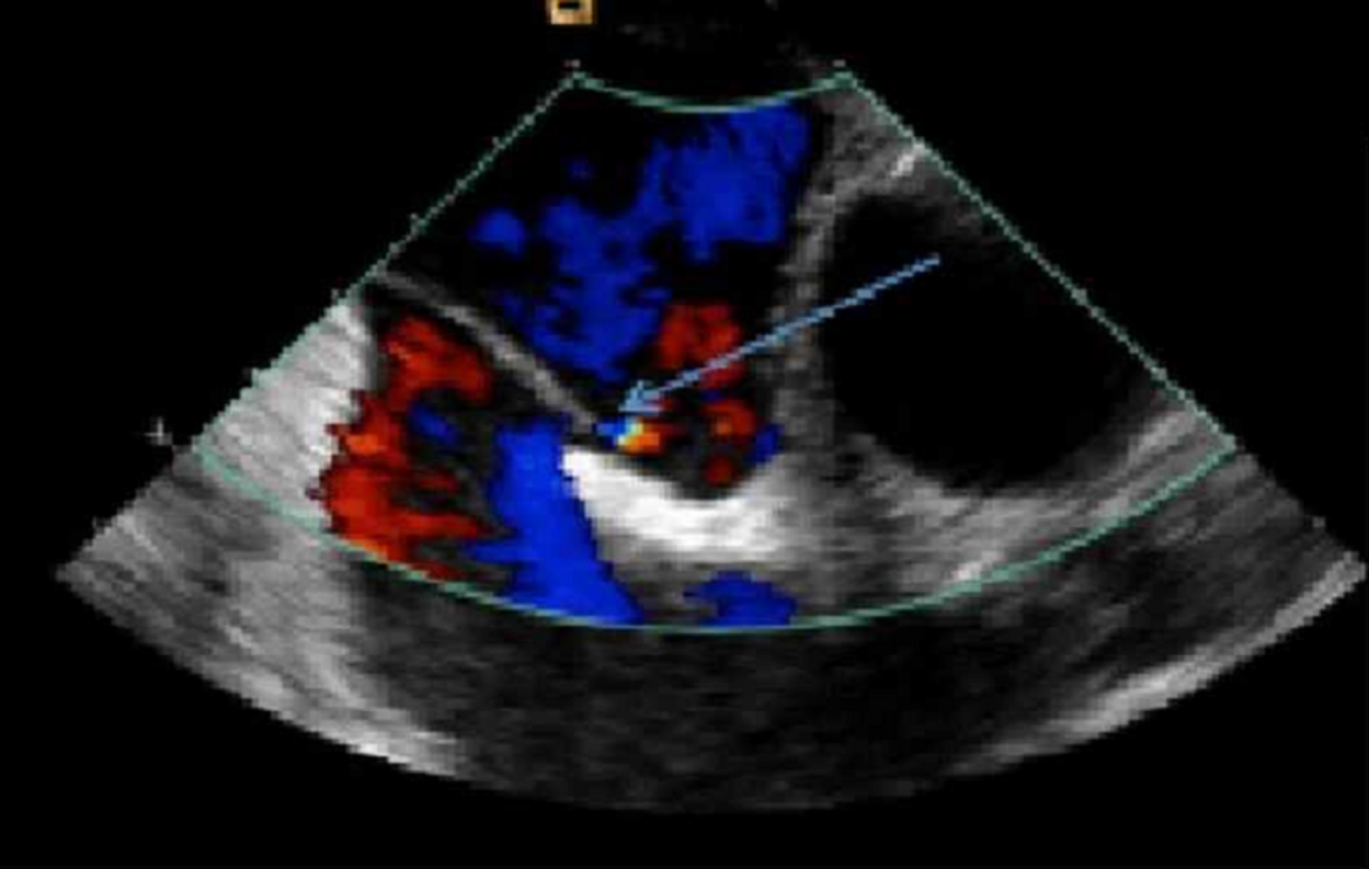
Transesophageal echocardiogram (TEE) with color Doppler showing a patent foramen ovale (PFO) with a right to left shunt
